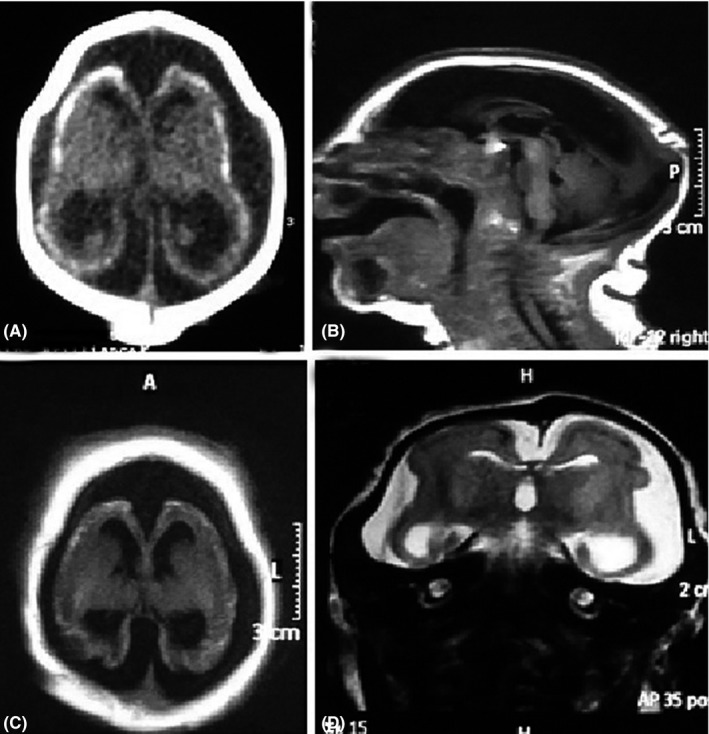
Severe microcephaly, cerebral atrophy with ventriculomegaly, and prominent cerebrospinal fluid space. Axial CT scan (A) shows extensive, punctate cortico‐subcortical calcifications, cerebral atrophy, and bone overlap. Sagittal T1 MR image (B) shows craniofacial disproportion, occipital protuberance with redundant posterior skinfolds, and a hypoplastic corpus callosum. The fossa is relatively preserved. Axial T1 (C) and coronal T2 (D) demonstrate the abnormal gyral pattern with diffuse undersulcated cortex
